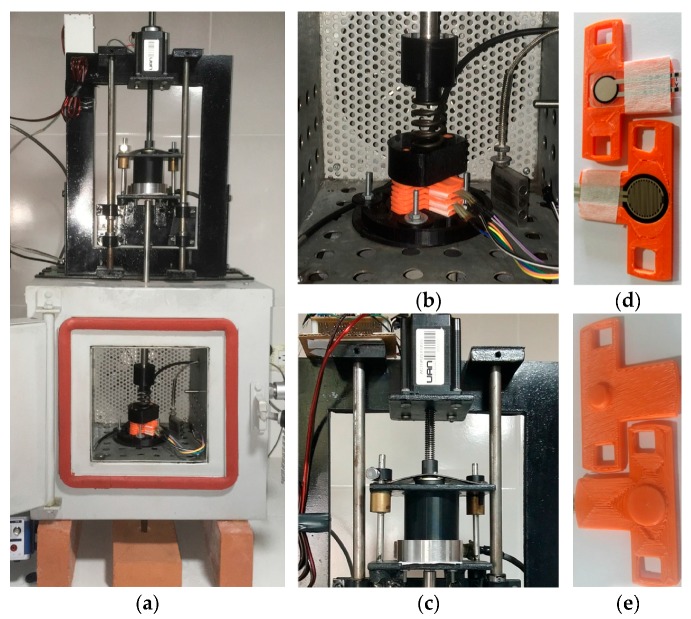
Mechanical setup for gathering sensor data. (a) Overview of the testbench; (b) Zoom-in picture depicting the sensors location inside the chamber and the spring for mechanical compliance; (c) Zoom-in picture showing the linear stepper motor and the LCHD-5 load cell, manufactured by Omega Engineering (Norwalk, CT, USA); (d) Top and (e) bottom views of the custom-made sensor holders and pucks, respectively; Sensors are harnessed using paper tape as in (d).
